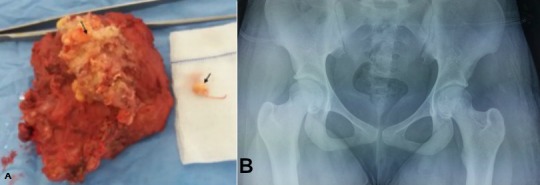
A) pièce d’exérèse de la tumeur; B) radiographie du bassin après 6 mois de l’intervention montre une disparition complète des dépôts calciques
